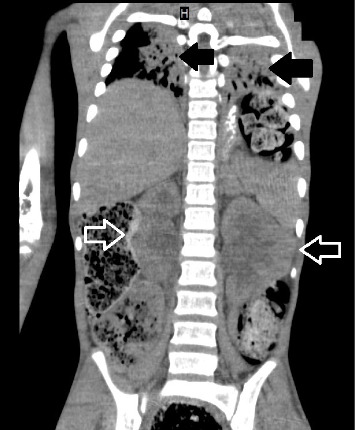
Coronal view of the abdominal CT scan, showing displacement of the liver and spleen, as well as significant dilatation of the renal pelvis and calyces due to bowel compression (white arrows). Note the restrictive bilateral lung compression with infiltrates (black arrows).
